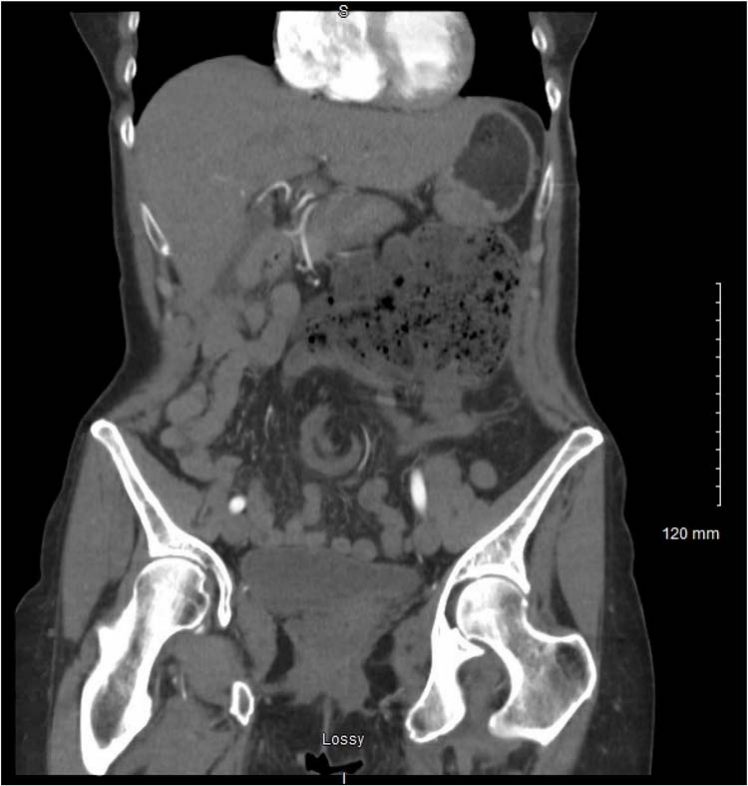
Coronal view of the patient’s CT abdomen and pelvis showing a central mesenteric swirl and adjacent fecalised bowel concerning for volvulus.
